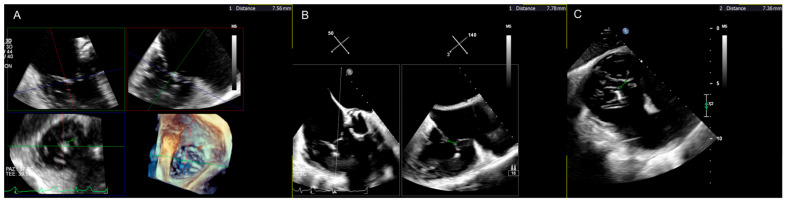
Coaptation gap measurement on a multiplanar reconstruction of a 3D trans-esophageal echocardiography dataset – red, blue and green lines represent orthogonal reference planes (A); biplane commissural view (B); transgastric en-face view (C).
